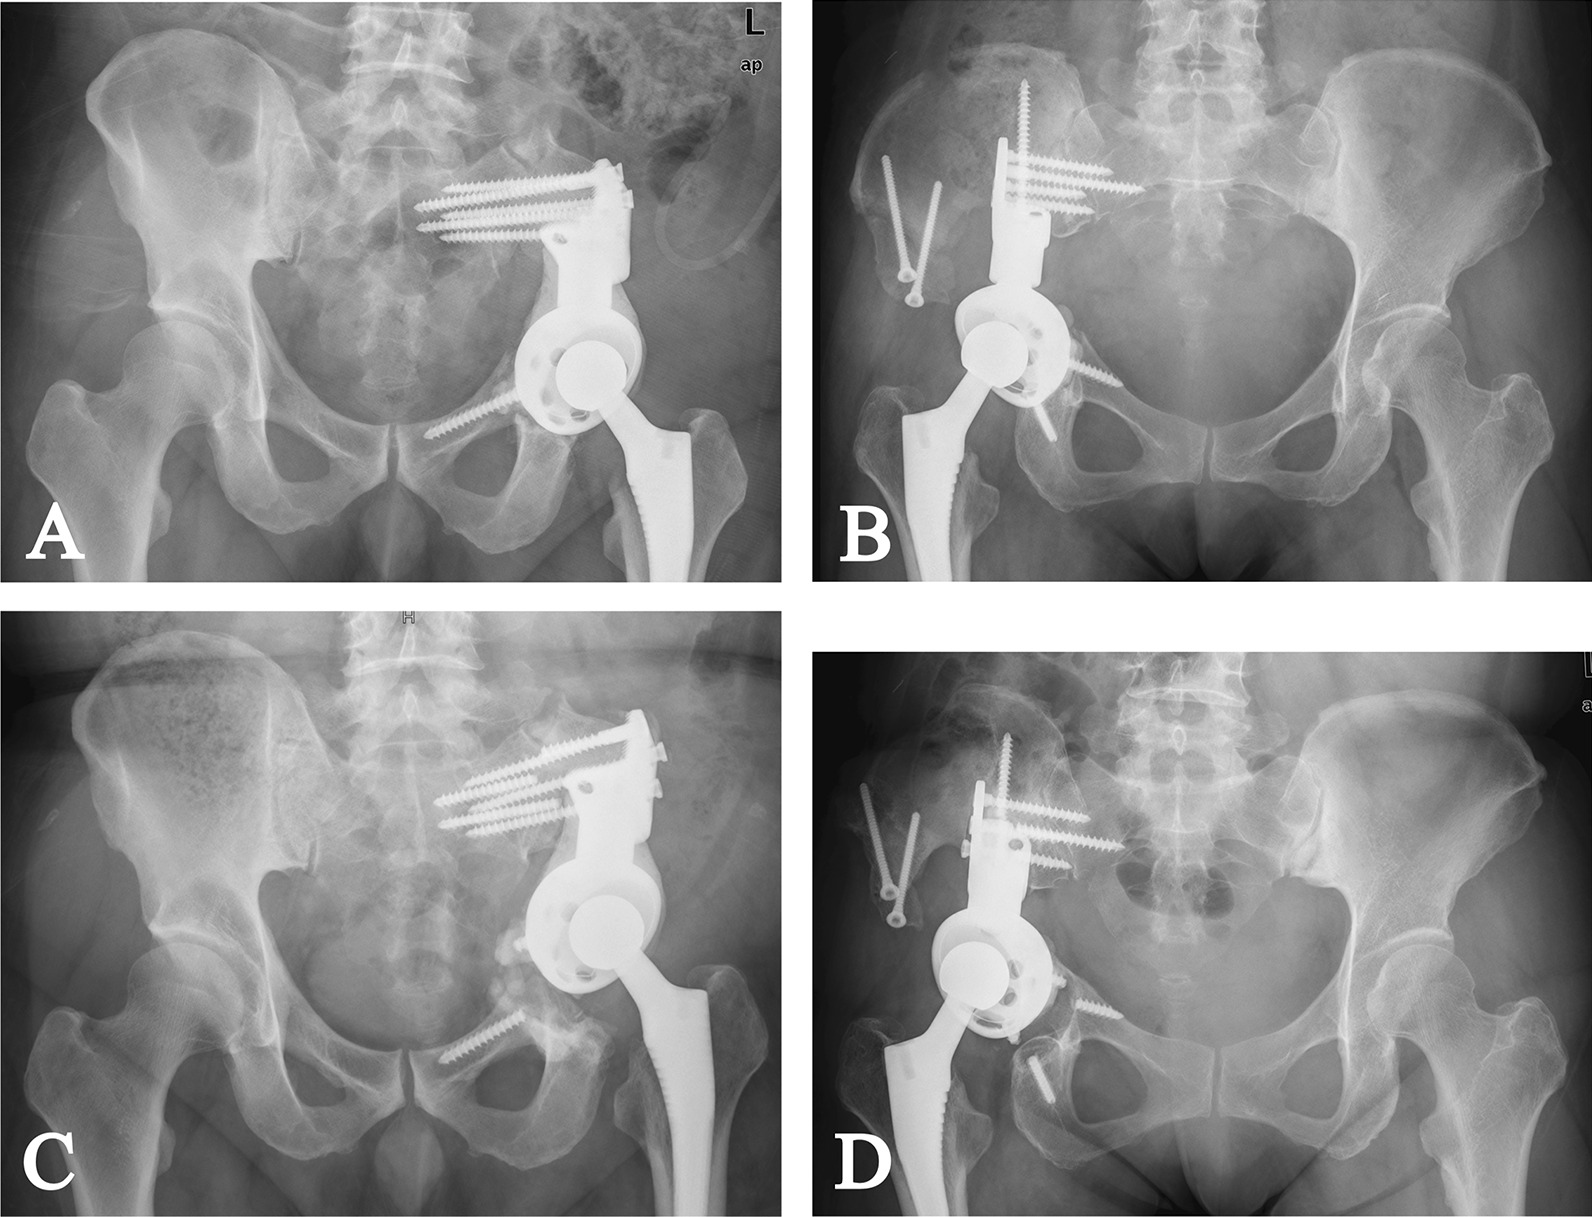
A, B Two AP plain radiographs of (A) a 60-year-old male with solitary plasmacytoma (Patient 1) and (B) a 43-year-old female with chondrosarcoma (Patient 2) after the primary surgery, which show reconstruction with modular hemipelvic endoprosthesis; C, D Two AP plain radiographs of (C) patient 1 and (D) patient 2 before revision, which show aseptic loosening, screw fracture and migration of modular hemipelvic endoprosthesis
